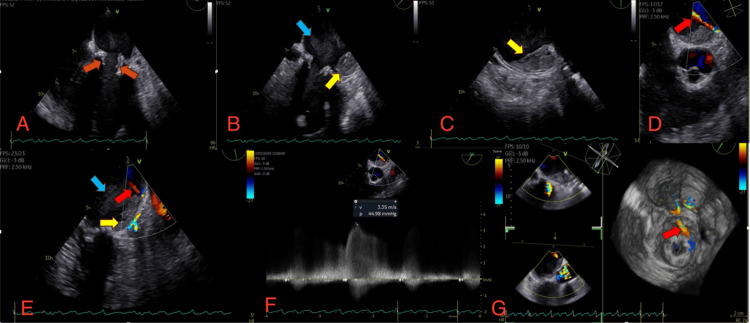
Transesophageal echocardiographic findings demonstrating left atrial thrombosis and coronary-cameral fistulous drainage into the left atrium. Panel A shows the Abbott bioprosthetic mitral valve with adequate leaflet opening (orange arrows). Panel B demonstrates dense spontaneous echocardiographic contrast within the left atrium (blue arrow) and an organized mural thrombus attached to the atrial wall (yellow arrow). Panel C further delineates the left atrial thrombus (yellow arrow). Panel D shows the coronary-cameral fistula (red arrow) adjacent to the aortic valve (center), with color Doppler demonstrating the fistulous tract draining into the left atrium. Panel E depicts the left atrium and left ventricle; the blue arrow indicates spontaneous echocardiographic contrast, the red arrow identifies the fistulous flow, and the yellow arrow indicates the atrial thrombus. Panel F demonstrates the continuous-wave Doppler profile of the fistulous flow velocity. Panel G shows three-dimensional transesophageal echocardiographic reconstruction, with the red arrow highlighting the fistulous tract draining into the left atrium.
